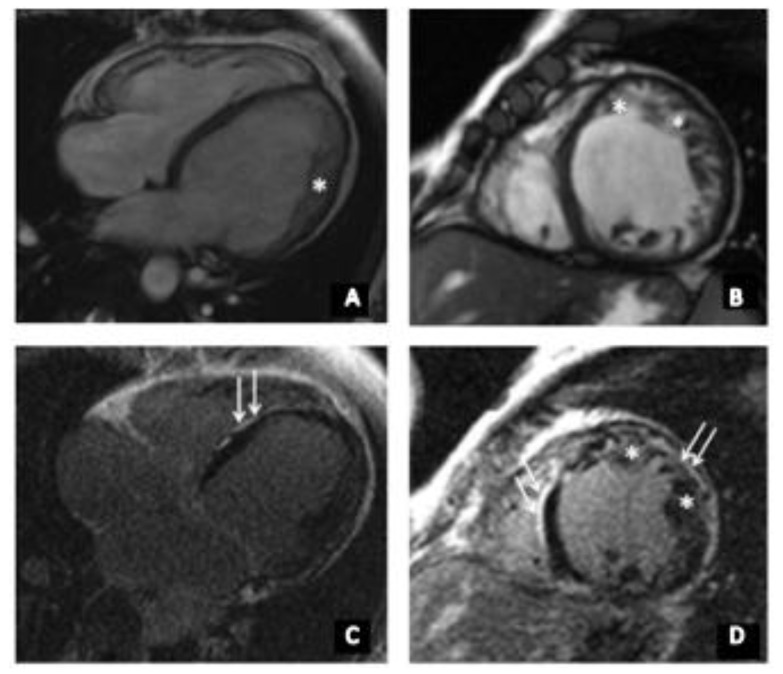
(A–D) cardiac MRI of index patient (II-2). * indicates non-compact myocardium (up to 18 mm). (A) Long-axis four-chamber projection, steady state free prcession (SSFP), and (B) short axis, SSFP, (C,D) Late gadolinium enhancement, areas of non-ischemic (subepicardial) contrast enhancement of left-ventricular walls are indicated with the white arrows.
